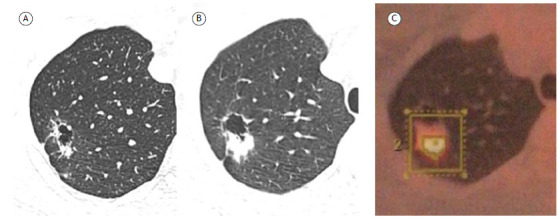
In A, axial unenhanced chest CT image showing a cystic lesion with irregularly thick walls in the right upper lobe of the lung and a small nodular component in the posterior region. In B, follow-up CT image obtained 4 months later, showing a significant increase in nodule size. In C, PET/CT image showing significant fluorodeoxyglucose uptake, with a standardized uptake value of 12.2.
